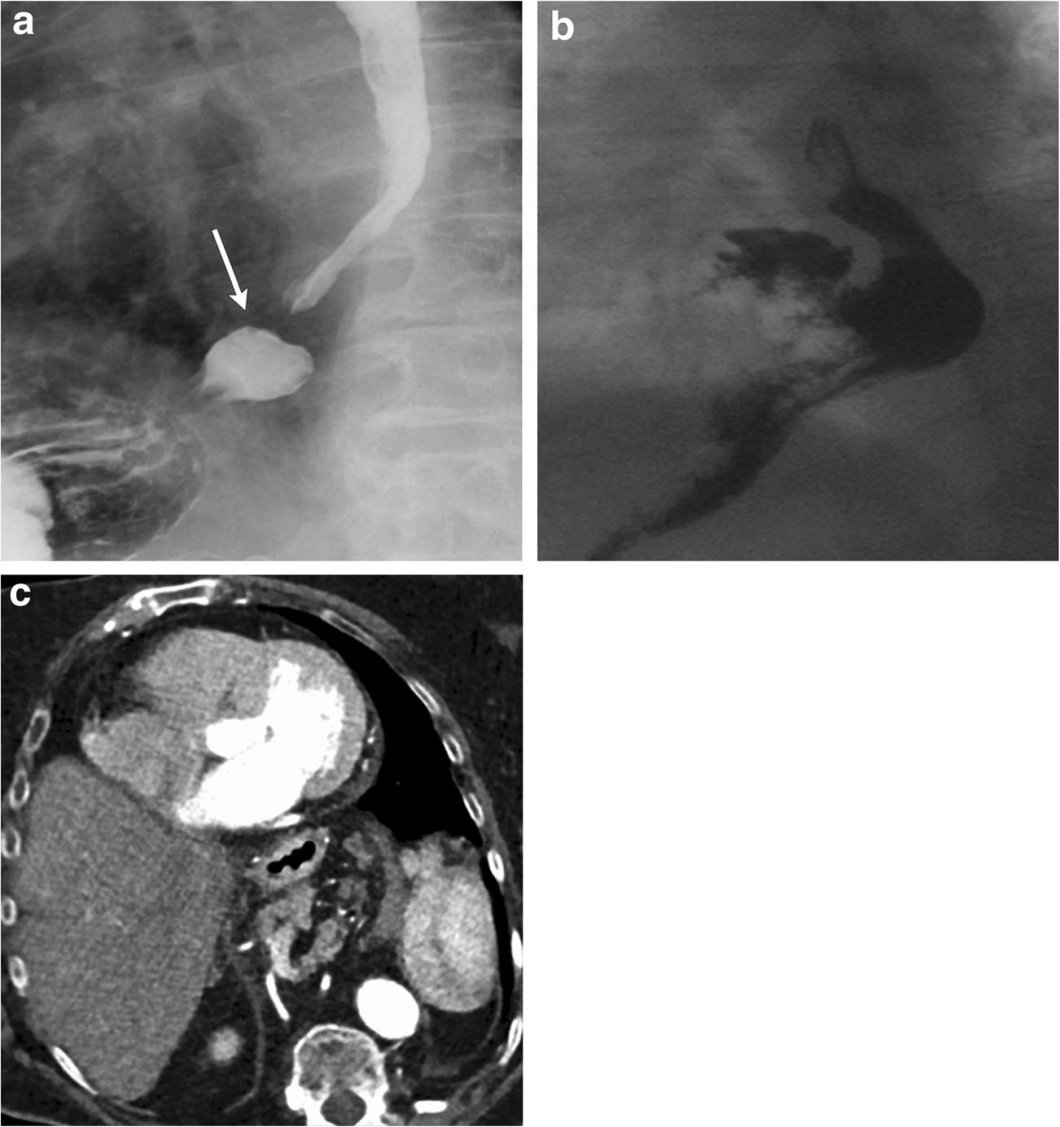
a A 79-year-old female with history of (h/o) heartburn on lying down after dinner. Left anterior oblique projection from the contrast oesophagogram identifies herniation of the gastro-oesophageal junction (white arrow) into the thorax; finding is consistent with type I or sliding hiatal hernia. b A 72-year-old male with morbid obesity and recurrent reflux pneumonitis. Lateral projection of a barium oesophagogram identifies intrathoracic herniation of the gastro-oesophageal junction with the gastric fundus, consistent with type III or mixed para-oesophageal hiatal hernia. c A 78-year-old female undergoing preoperative evaluation for transcatheter aortic valve replacement. Axial contrast CT identifies a large hiatal hernia containing stomach, large bowel, splenic vessels and pancreas in the hernia sac, compatible with a type IV hiatal hernia (Movie 2)
